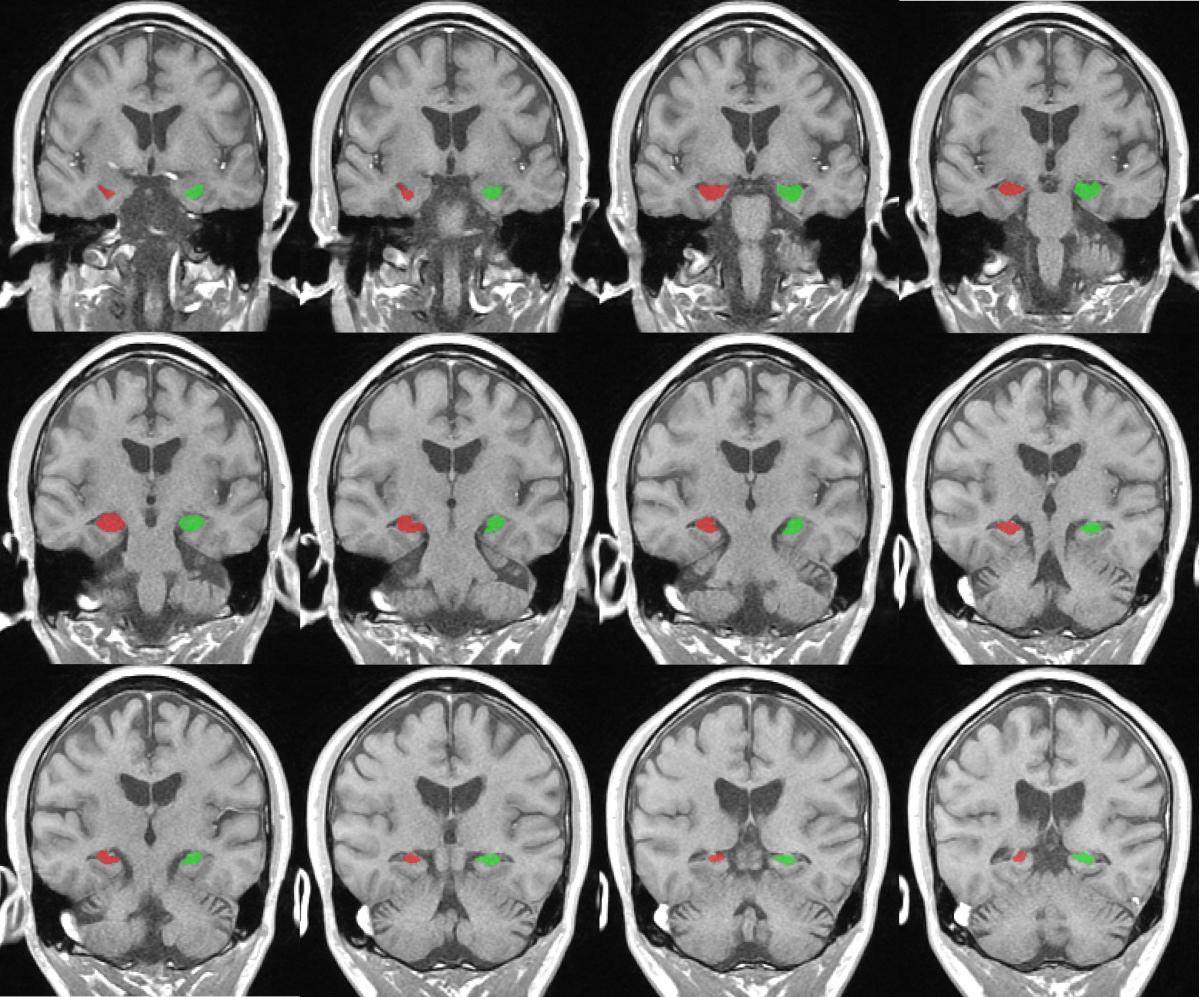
Representative example of right (red) and left (green) hippocampus segmentation and volume calculation on T1-weighted images in ITK-snap software.
